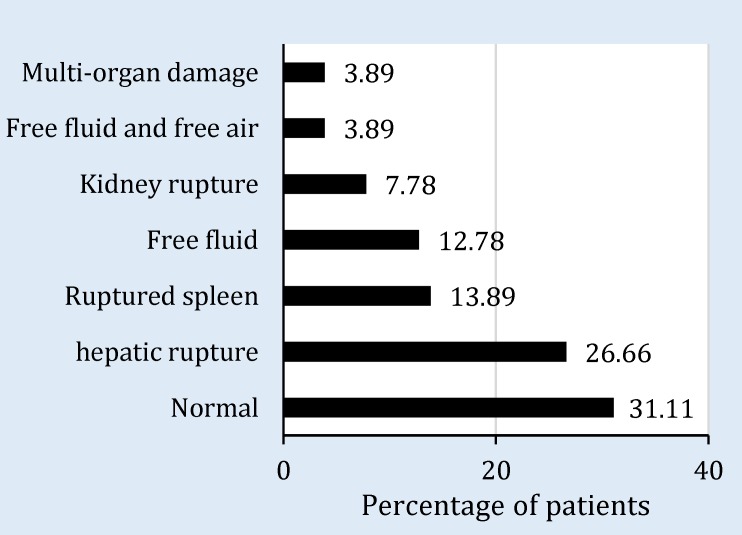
Abdominopelvic computed tomography scan findings of FAST positive patients
